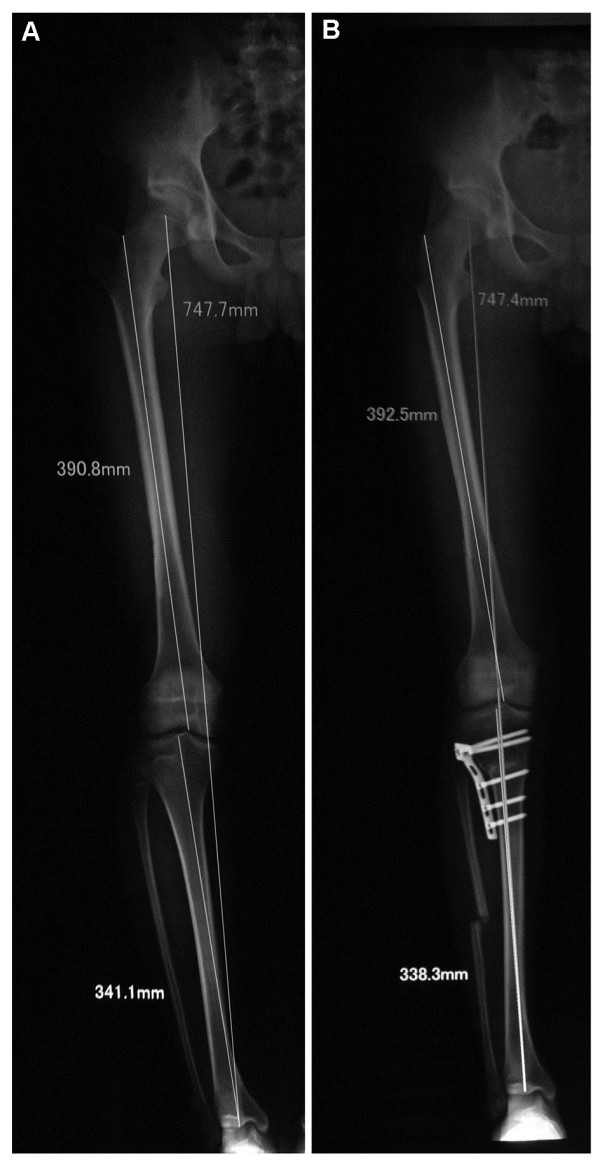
FTA correction by a modified CWHTO. (A) Preoperative; (B) 12 weeks postoperative. Full-length AP radiographs of a case of osteotomy showing correction of mechanical axis of lower limbs (Mikulicz line).
